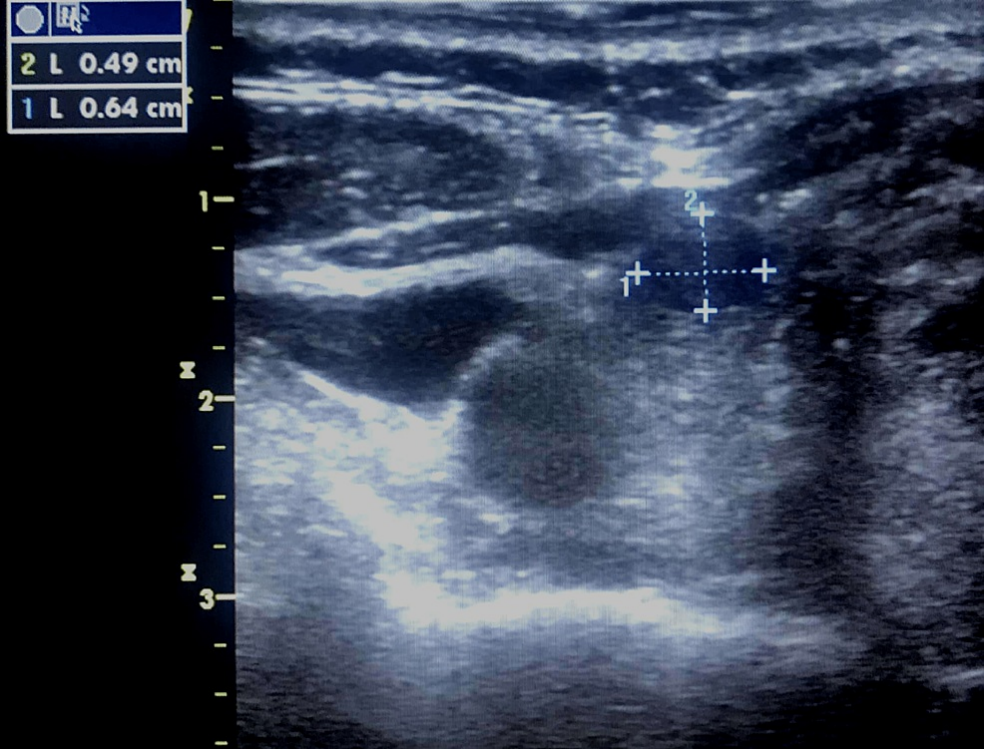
Neck ultrasound showing a 4 x 6 mm (0.49 x 0.64 cm) thyroid cystic mass, compatible with shrinkage of the previously treated thyroid nodule
